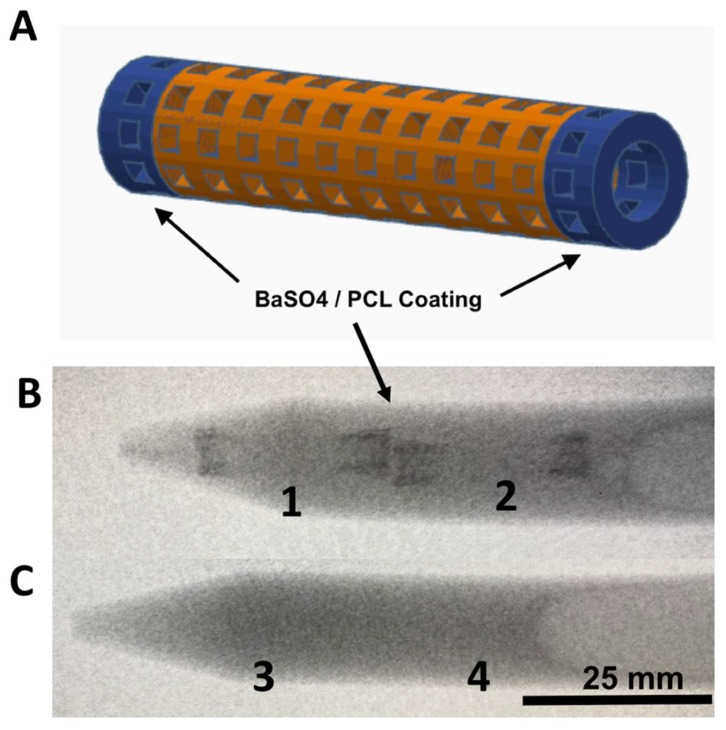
Images of CAD stent in (A), X-ray images of 3D printed XL-PVA stents with barium coated tips (B; 1 and 2), and X-ray image of control XL-PVA stents (C; 3 and 4). Black arrows point to regions of interest containing barium sulfate (scale bar = 25 mm).
